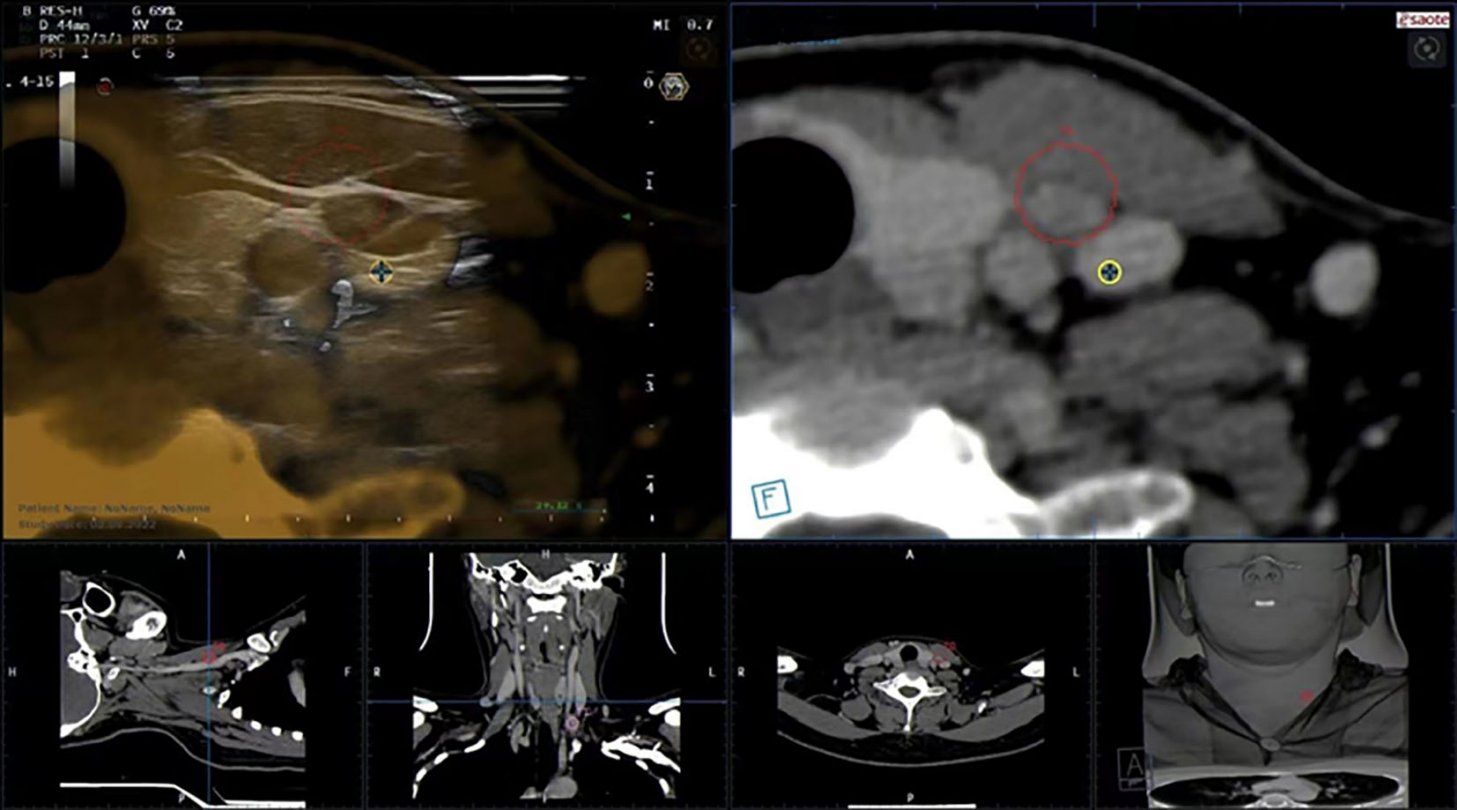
US-CT fusion imaging shows that the LN marked after image fusion was in the red circle, and LN metastasis in PTC was confirmed by FNAC.
